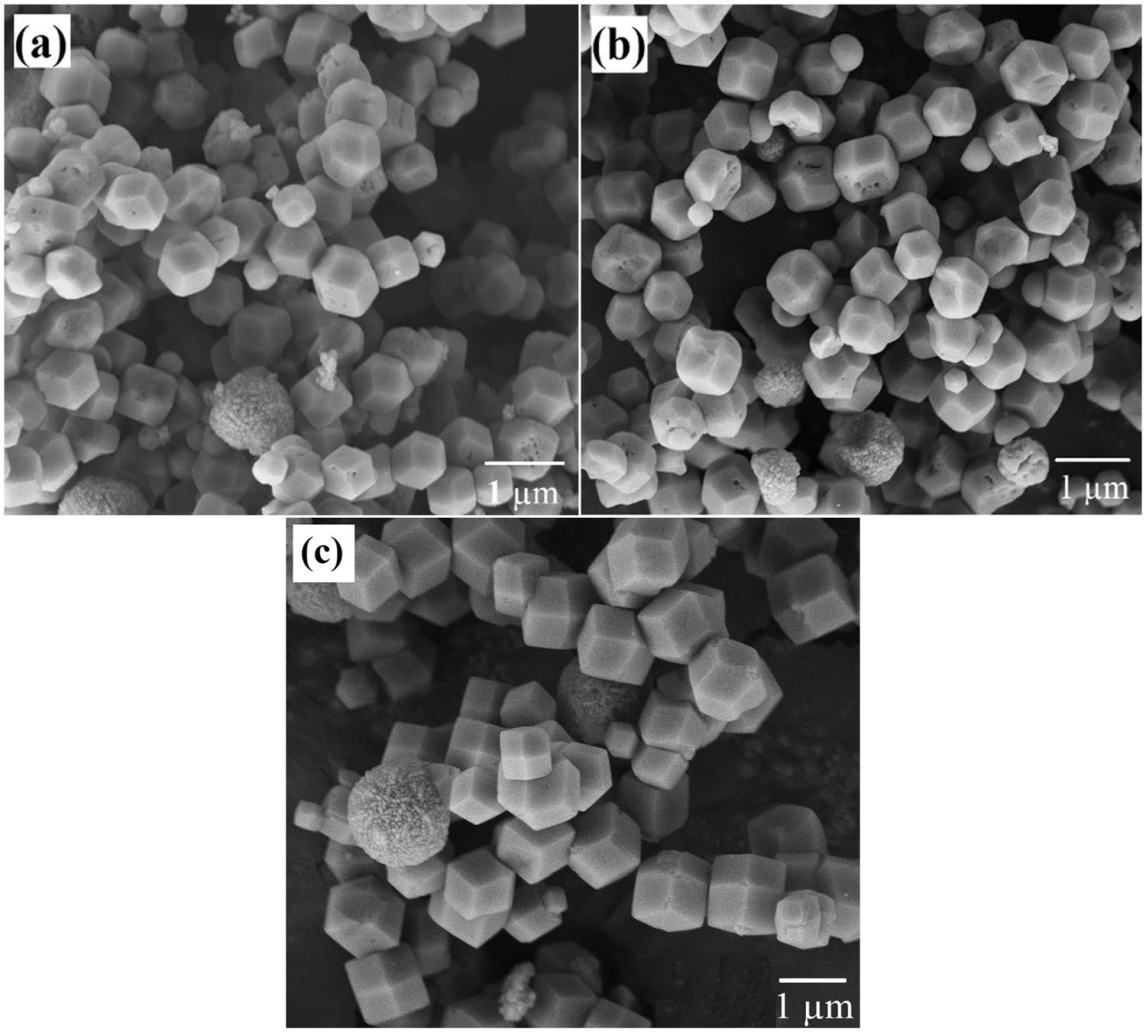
FESEM images of the samples pretreated with varied ultrasonic irradiation time of (a) 30 minutes (S4), (b) 60 minutes (S5), (c) 120 minutes (S6) and followed by 2 days of hydrothermal heating.
